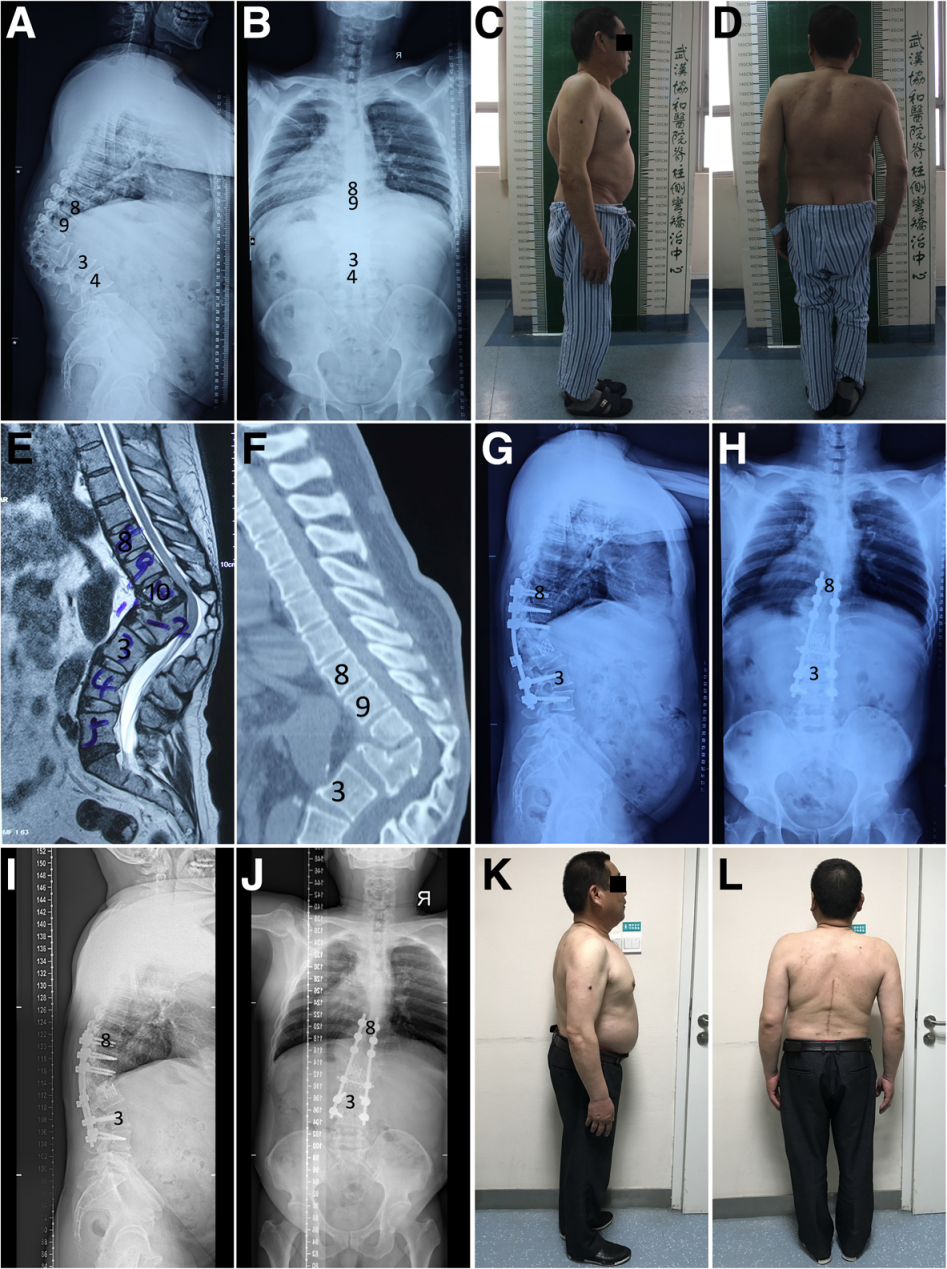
Standing radiographs and images of a 50-year-old male patient with severe post-tubercular kyphosis. a, b Preoperative lateral and antero-posterior radiographs, demonstrating severe angular kyphosis, with preoperative kyphotic angle 86° and sagittal vertical axis 45 mm. c, d Preoperative appearance in side and posterior view. e Preoperative magnetic resonance imaging scanning demonstrates stenosis adjacent to the angular kyphosis and severe spinal compression. f Preoperative computed tomography scanning demonstrates severe angular kyphosis and “buckling collapse.” g, h Postoperative lateral and antero-posterior radiographs, showing correction of thoracolumbar kyphosis after PVCR of T11, T12, and L1, with postoperative kyphotic angle 27° and sagittal vertical axis 33 mm. i, j Postoperative lateral and antero-posterior radiographs, showing excellent correction of thoracolumbar angular kyphosis and osteotomy site fusion at the 24-month follow-up, with kyphotic angle 29° and sagittal vertical axis 42 mm. k, l Postoperative appearance in side and posterior view
